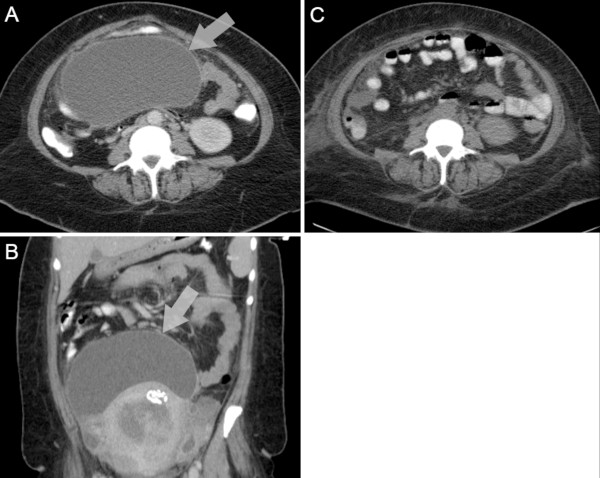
Computed tomography scans showing a large abscess within the patient’s pelvis that was culture positive for S. agalactiae. Transverse ( A) and coronal ( B) contrast-enhanced computed tomography scans of the patient showing a large abscess within her pelvis extending to the right adnexa (arrow) that was culture positive for *S. agalactiae.* Near complete resolution was achieved ( C) one day after fluoroscopic-guided abscess drainage.
